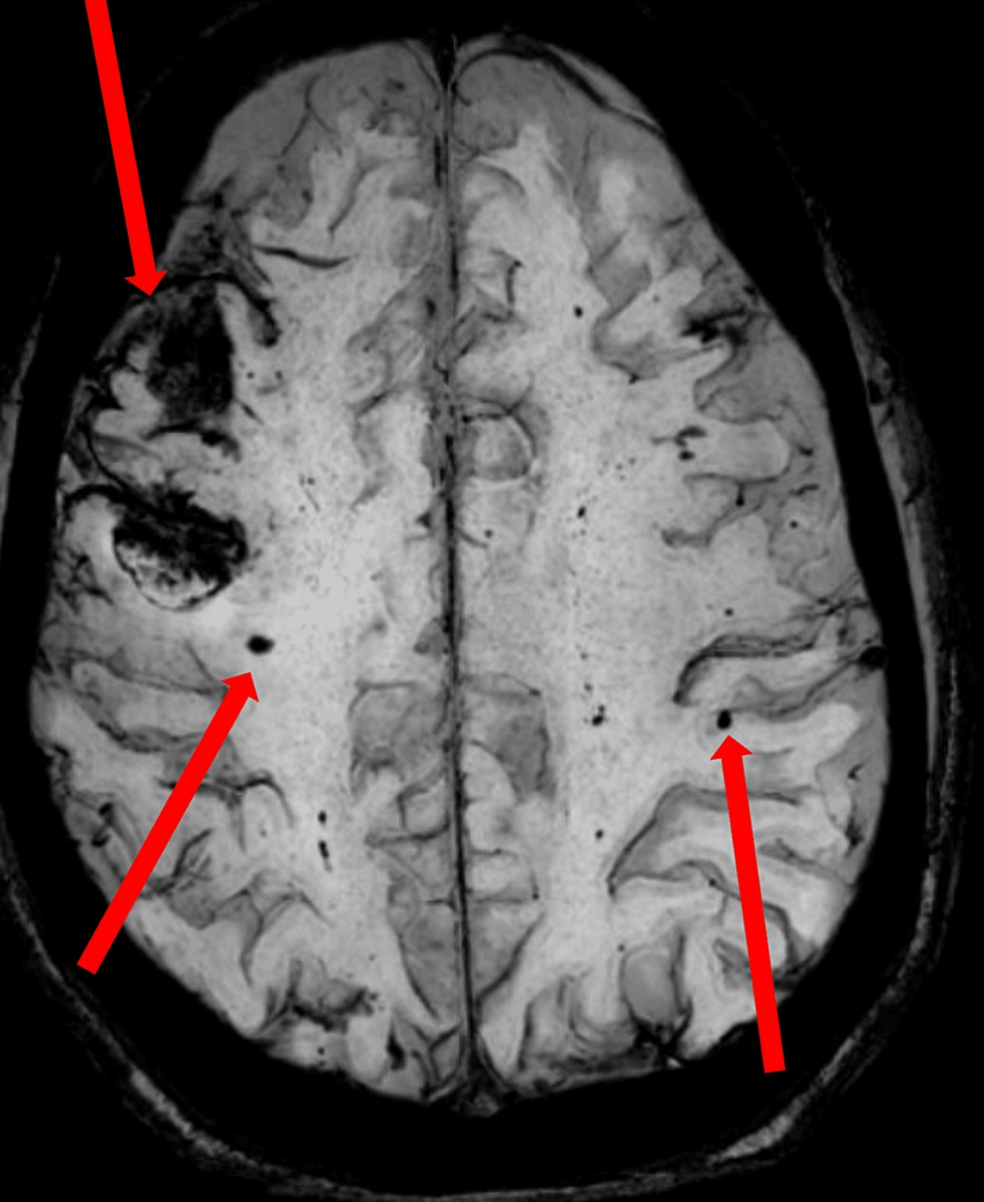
Follow-up MRI susceptibility-weighted imaging shows numerous tiny parenchymal bleeds, infarcts, and extra-axial hemorrhages (arrows).
